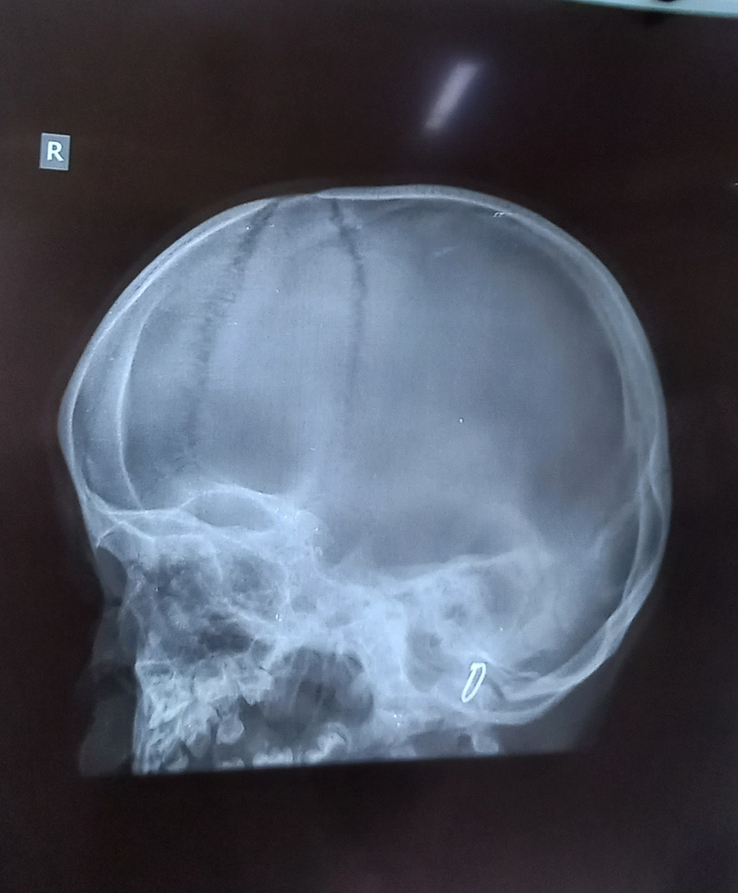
X-ray skull showing sutural diastasis.
